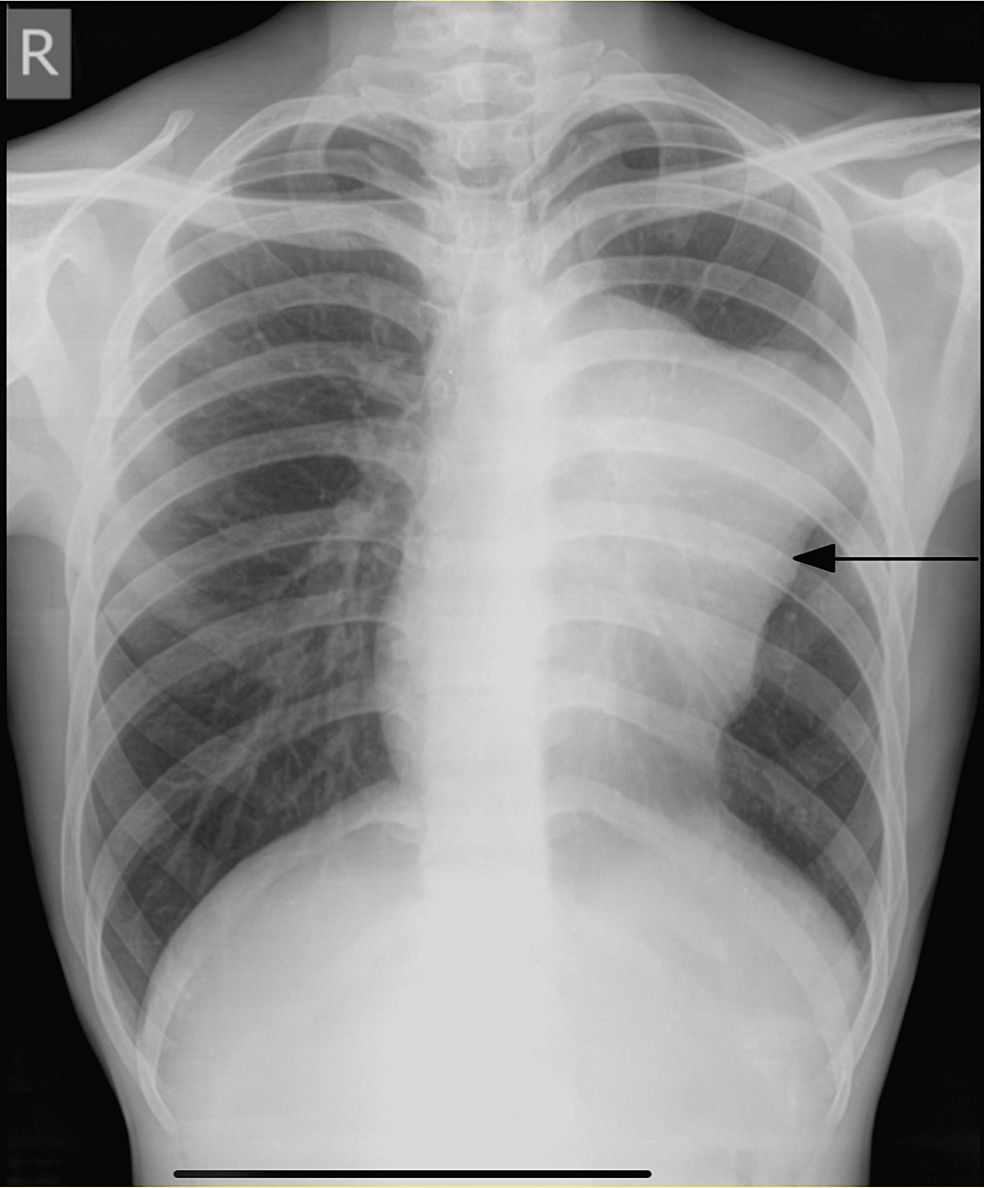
Preoperative chest x-ray showing a left-sided opacity
